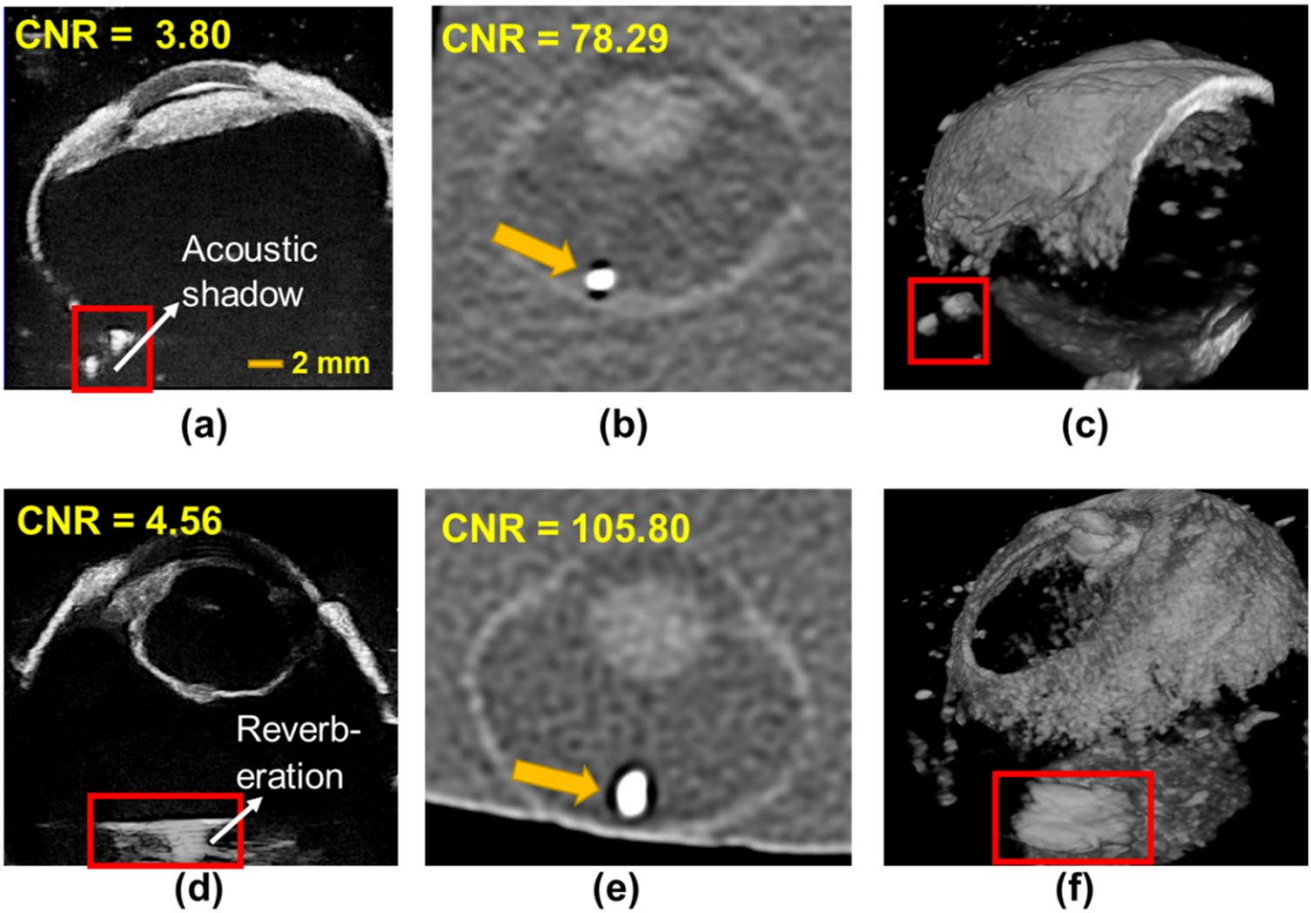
Visualization of metal and glass IOFB in 3D-UBS and CT. (a)–(b) and (d)–(e) show 2D views of the same metal and glass IOFB using 3D-UBS (window/level = 255/127) and CT (window/level = 450/75), respectively. (c) and (d) show 3D volume rendering of the IOFBs in 3D-UBS.
